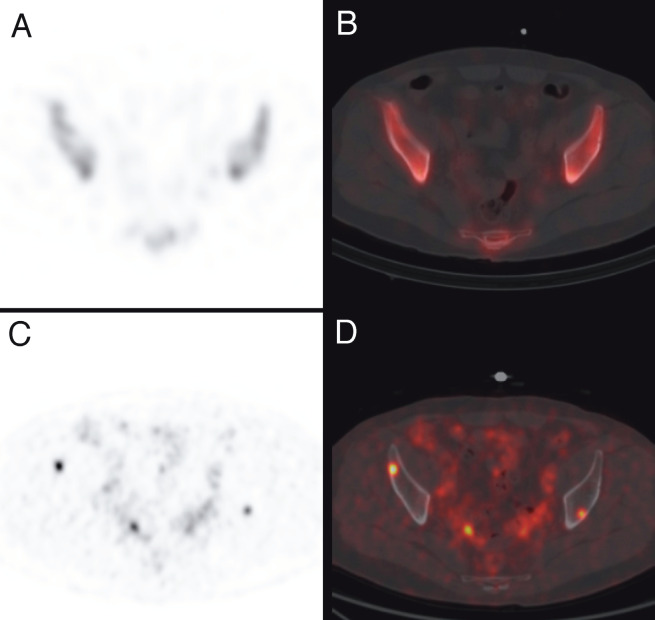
Tc-99m MDP bone scintigraphy SPECT (A), fused SPECT/CT (B), Ga-68 PSMA axial PET (C), and fused PET/CT (D) images of the same prostate cancer patient. Ga-68 PSMA PET shows additional bone metastasis that are false negative on bone SPECT and SPECT/CT.
